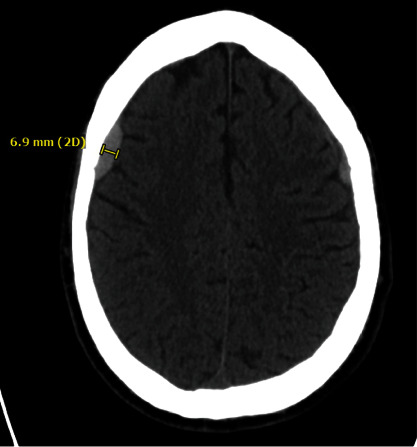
CT of left SDH at time of presentation in the emergency department.
